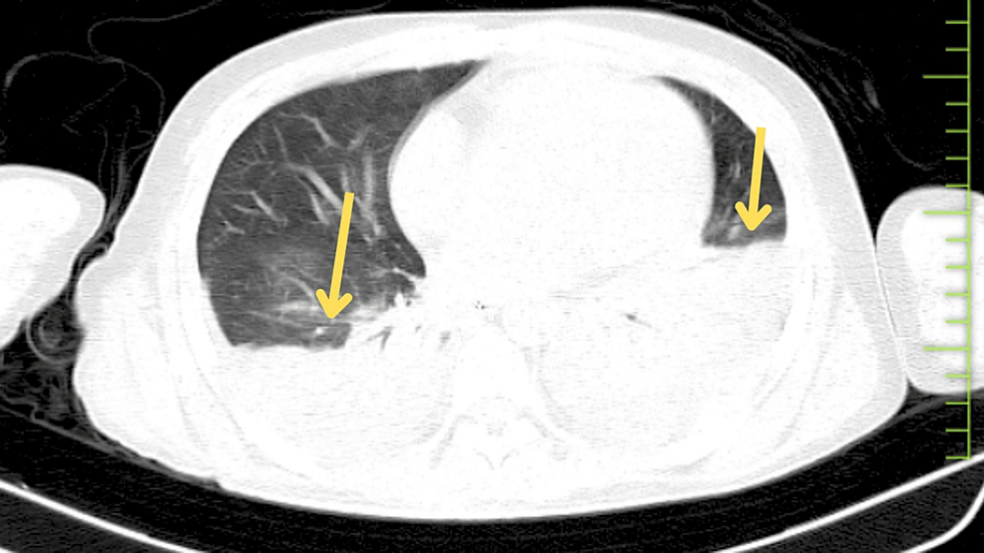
HRCT thorax HRCT: high-resolution computed tomography. Yellow arrow points toward bilateral pleural effusion (left>right) with subsegmental atelectasis.
